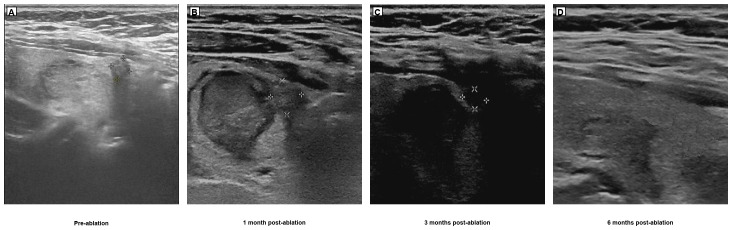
Representative ultrasound follow-up of a capsule-adjacent papillary thyroid carcinoma treated with radiofrequency ablation. (A) Pre-ablation image showing the index tumor. (B) One month after ablation. (C) Three months after ablation, with progressive reduction of the ablation zone. (D) Six months after ablation, with complete tumor disappearance of the treated tumor.
